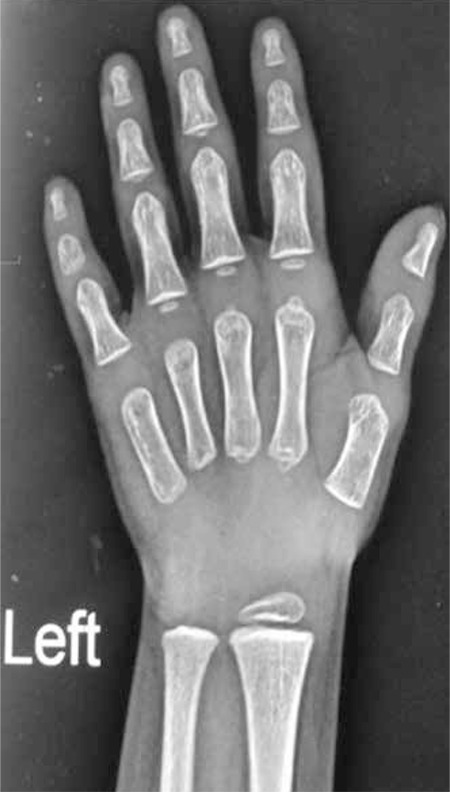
X-ray of the left hand and wrist showing a bone age of less than one year
